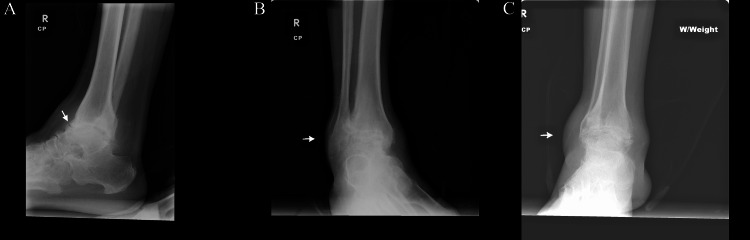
Radiographs of the right ankle 16 years before most recent hospital admission. Lateral view (A), mortise view (B), and anteroposterior view (C) with weight bearing demonstrate marked soft tissue swelling over the ankle, osteophytes, uniform joint space narrowing of the ankle and subtalar joints, and partial fusion of the right ankle joint as well as small calcaneal enthesophytes at the insertion of the Achilles tendon.
